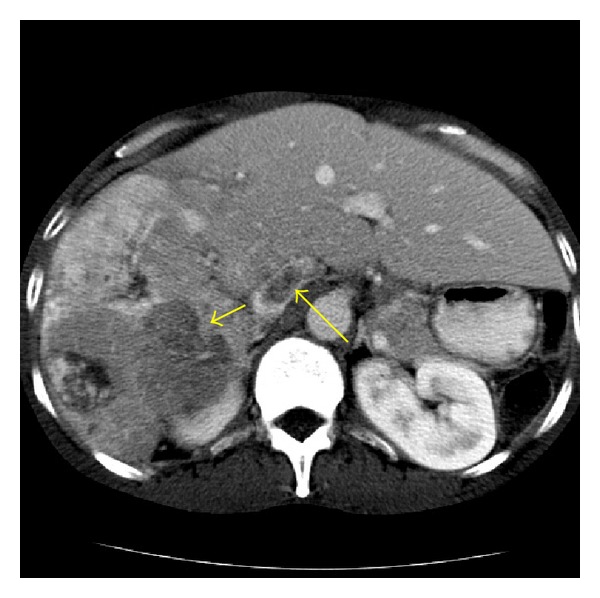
HCC invading the inferior vena cava. Contrast enhanced axial CT scan shows a central filling defect in the inferior vena cava (long arrow). Large peripheral enhancing hepatocellular carcinoma compressing the right kidney is also shown (short arrow).
